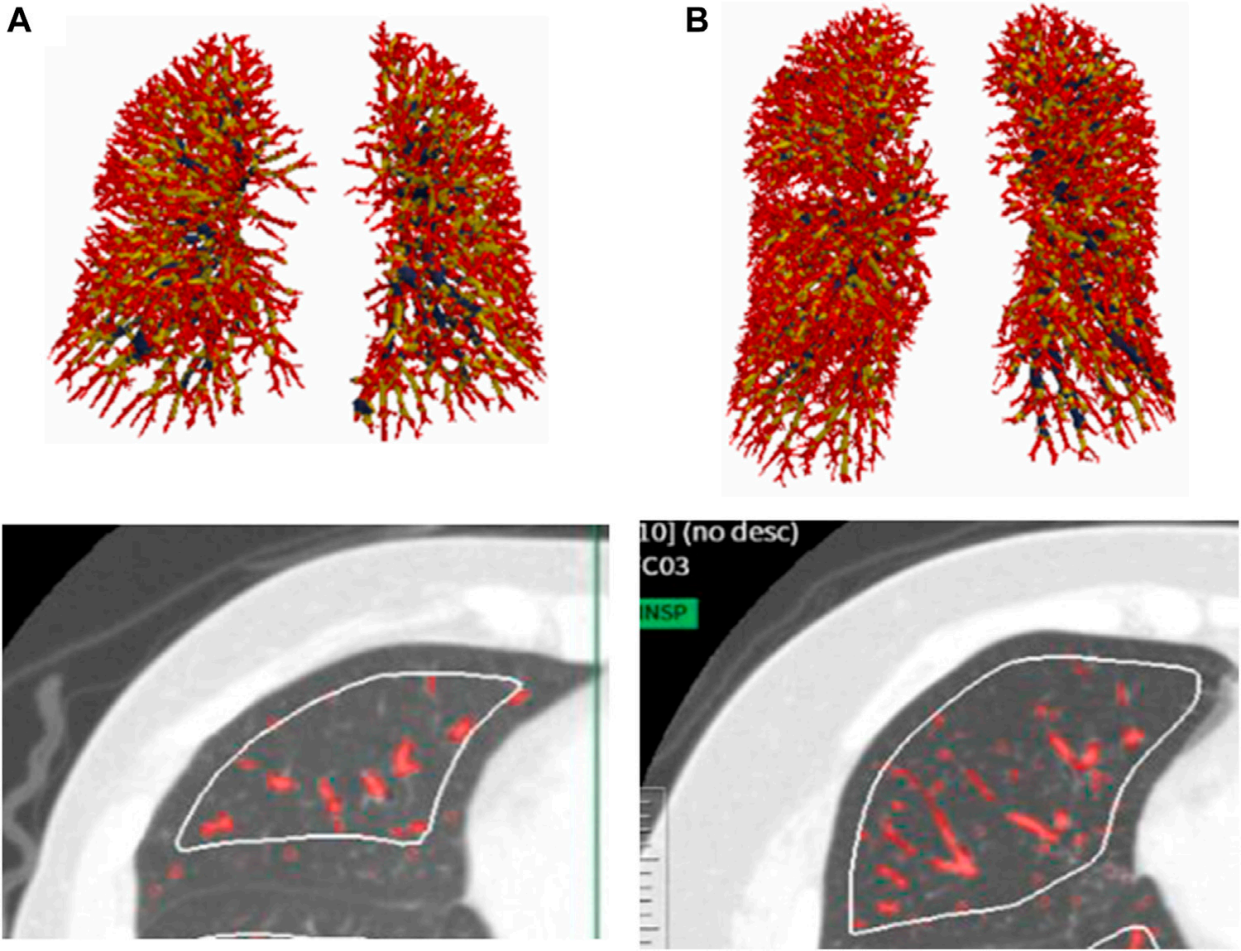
Representative three-dimensional imaging and axial CT images in two female patients. One with low VA/TLC (60.9%) had 1943 counts of BV5 number, and 109.8 mL of BV5 volume (A), while another with high VA/TLC (83.%) had 2812 counts of BV5 number, and 156 mL of BV5 volume (B).
